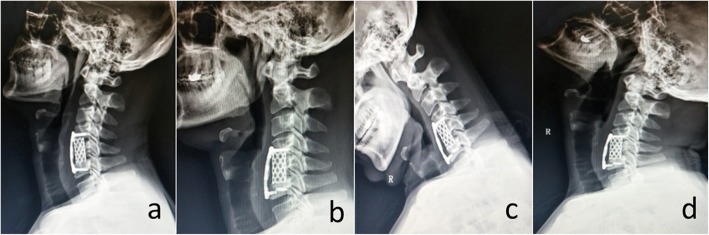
a A 43-year-old male patient underwent C5 corpectomy and fusion. b Lateral radiograph showed screw breakage while the subsidence was not obvious 1 month after surgery. c, d Flexion–extension lateral radiographs demonstrated poor bone fusion which may result to reconstruction failure
